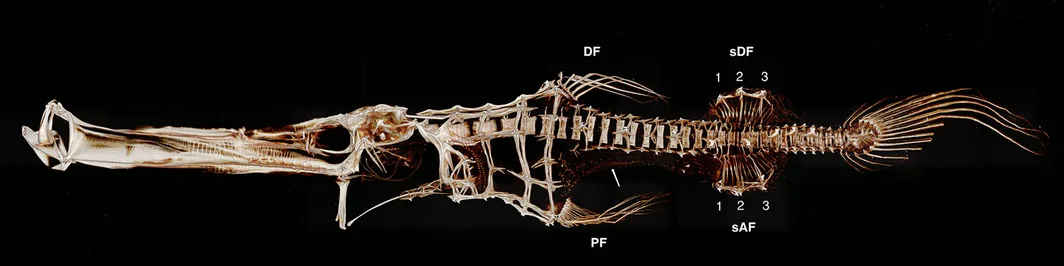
Micro‐CT reconstruction (lateral view) of CAS 240194. DF, dorsal‐fin; PF, pectoral‐fin; sAF, soft anal‐fin; sDF, soft dorsal‐fin. Numbers 1, 2 and 3 indicate the respective pterygiophores supporting the soft dorsal‐ and anal‐fins. White arrow indicates a series of small ossicles ventral to the vertebral column between the pretrunk and soft‐fins, forming a single layer of small elements in this region.
